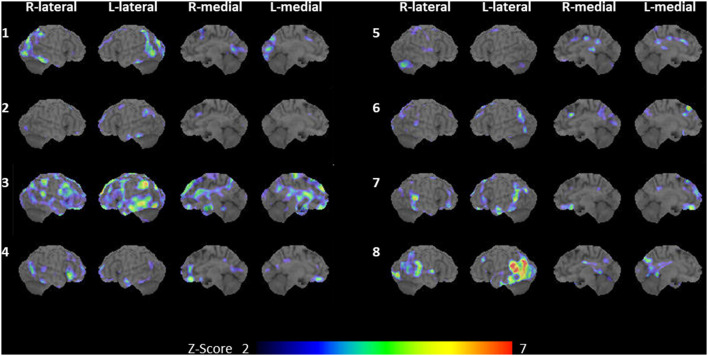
Brain SPECT. IMP-SPECT analyzed with 3D stereotactic surface projections revealed patterns of significant hypoperfusion in each of the eight cases with an aphasic MCI-LB. Case numbers are shown to the left of each set of images. Cases 1–5: progressive anomic aphasia; cases 6–8: logopenic progressive aphasia. L, left; R, right; aphasic MCI-LB, aphasic mild cognitive impairment with Lewy bodies; IMP-SPECT, *N*-isopropyl-p-[^123^I] iodoamphetamine single-photon emission computed tomography.
